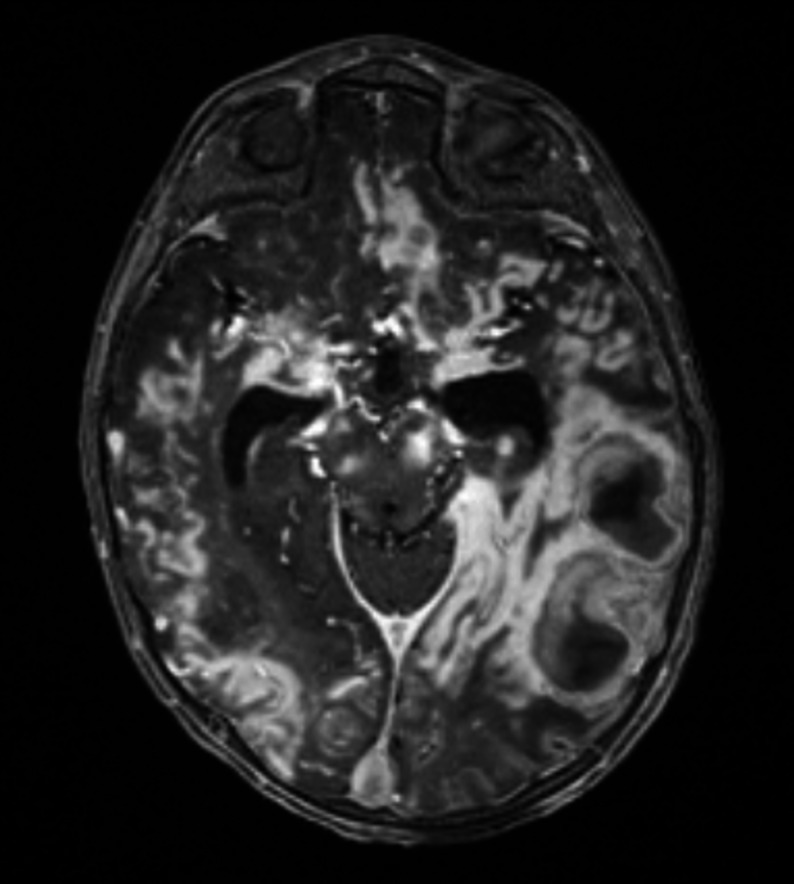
Post-contrast T1-weighted image (B) shows prominent bright enhancement of the cerebral parenchyma with characteristic perivascular and cortical “ribbon-like” involvement
